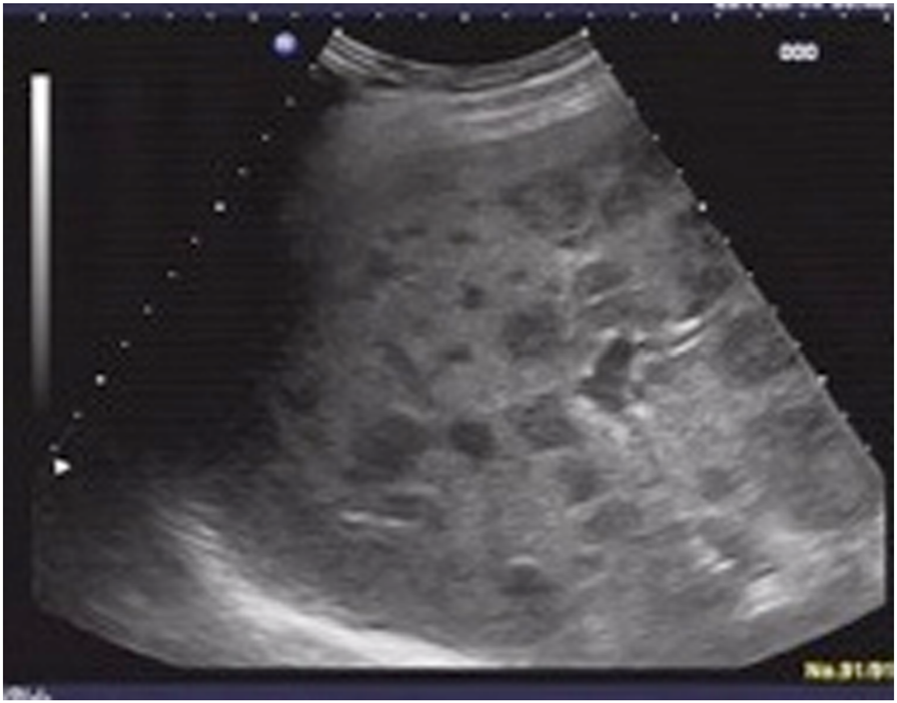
Liver ultrasound. Multiple liver lesions.
